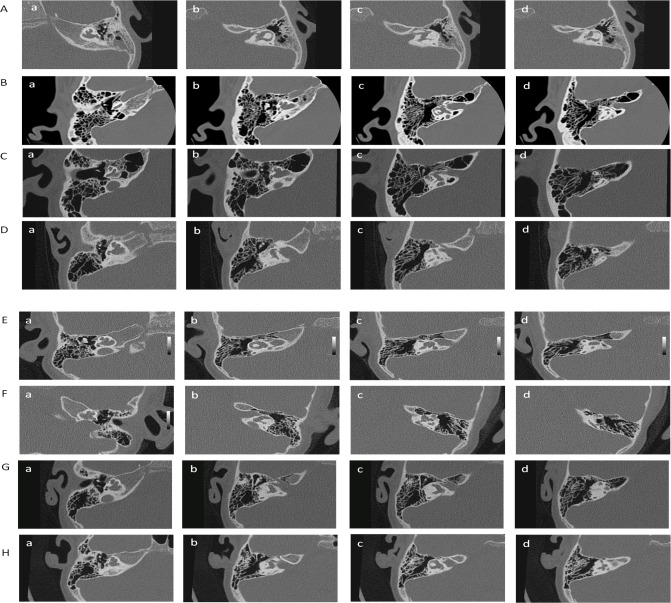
CT of temporal bones in the probands from families with POU3F4 mutations: A- p.Gln27*; B—p.Ala116fs141*; C-p.Glu187*; D- p.Leu208*; E- p.Leu217*; F- p.Gln275*; G- p.Gln306*; H- p.Val324Asp. CT of temporal bone displays widened internal auditory canal (b, c) and inner ear malformations with leading feature of underdeveloped cochlear modiolus with complete absence of its base (a, b). Other features are: distorted vestibule (b, c) with small outpouchings (c, d), widened vestibular aqueduct (c), irregular semicircular ducts (c, d), The interscalar septa are preserved (a, b).
